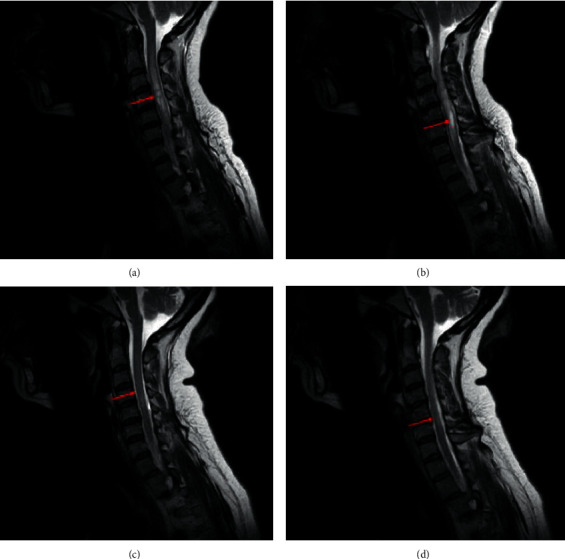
MRI of the cervical spinal cord-sagittal T2-weighed sequences ((a)–(d)). ((a), (b)) Sequences from February 2019 show an expansion of the cervical spinal cord involving a long segment from C1 through C7. ((c), (d)) Sequences from June 2020 show interval minimization in hyperintensity and swelling within the cervical spinal cord, and when compared to (a) and (b), no enhancing lesions are noted.
